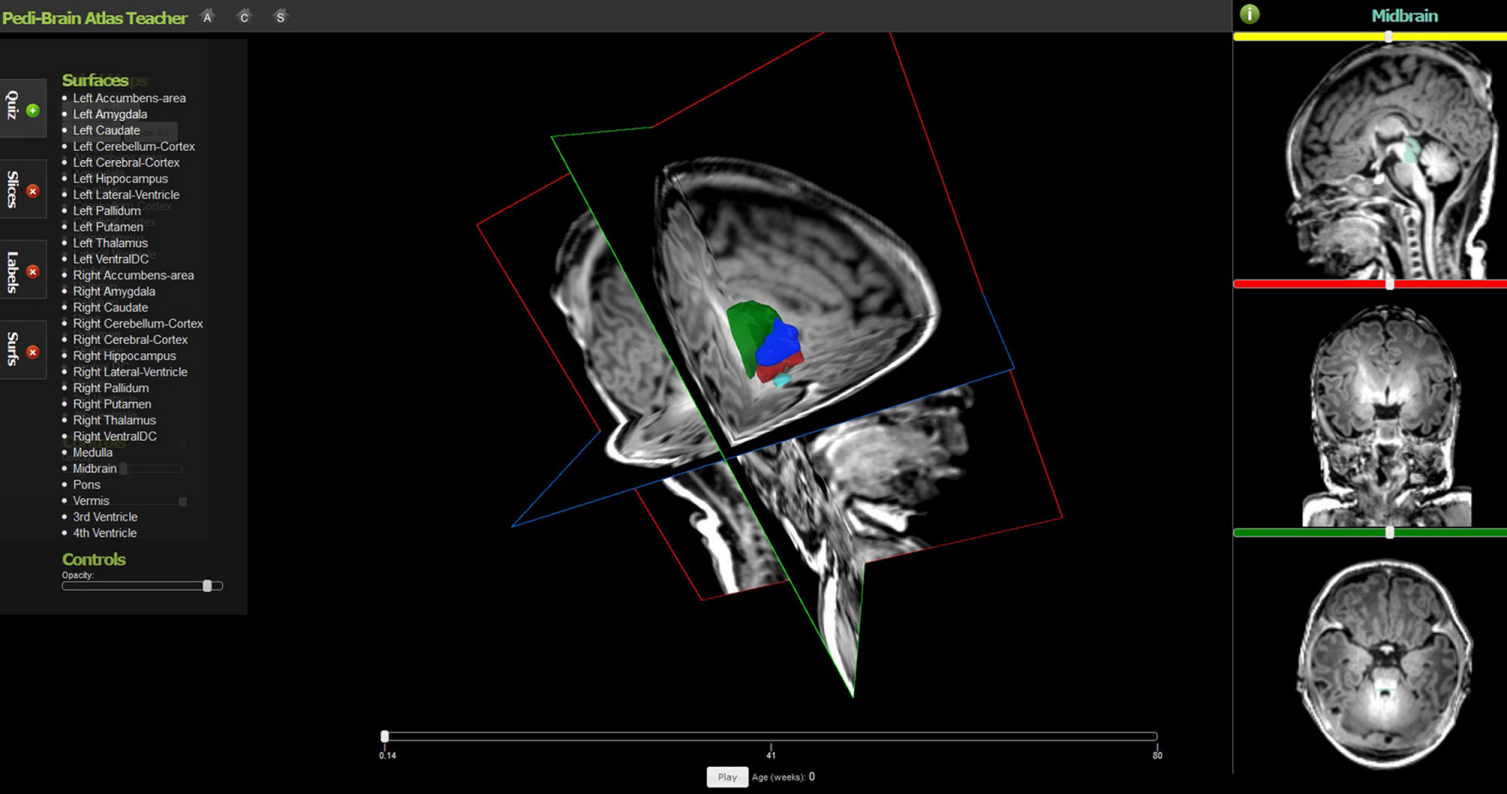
The Pedi-Brain Atlas Teacher, an interactive visualization tool for pediatric brain tumors on MRI (available at: http://fnndsc.github.io/babybrain/).
